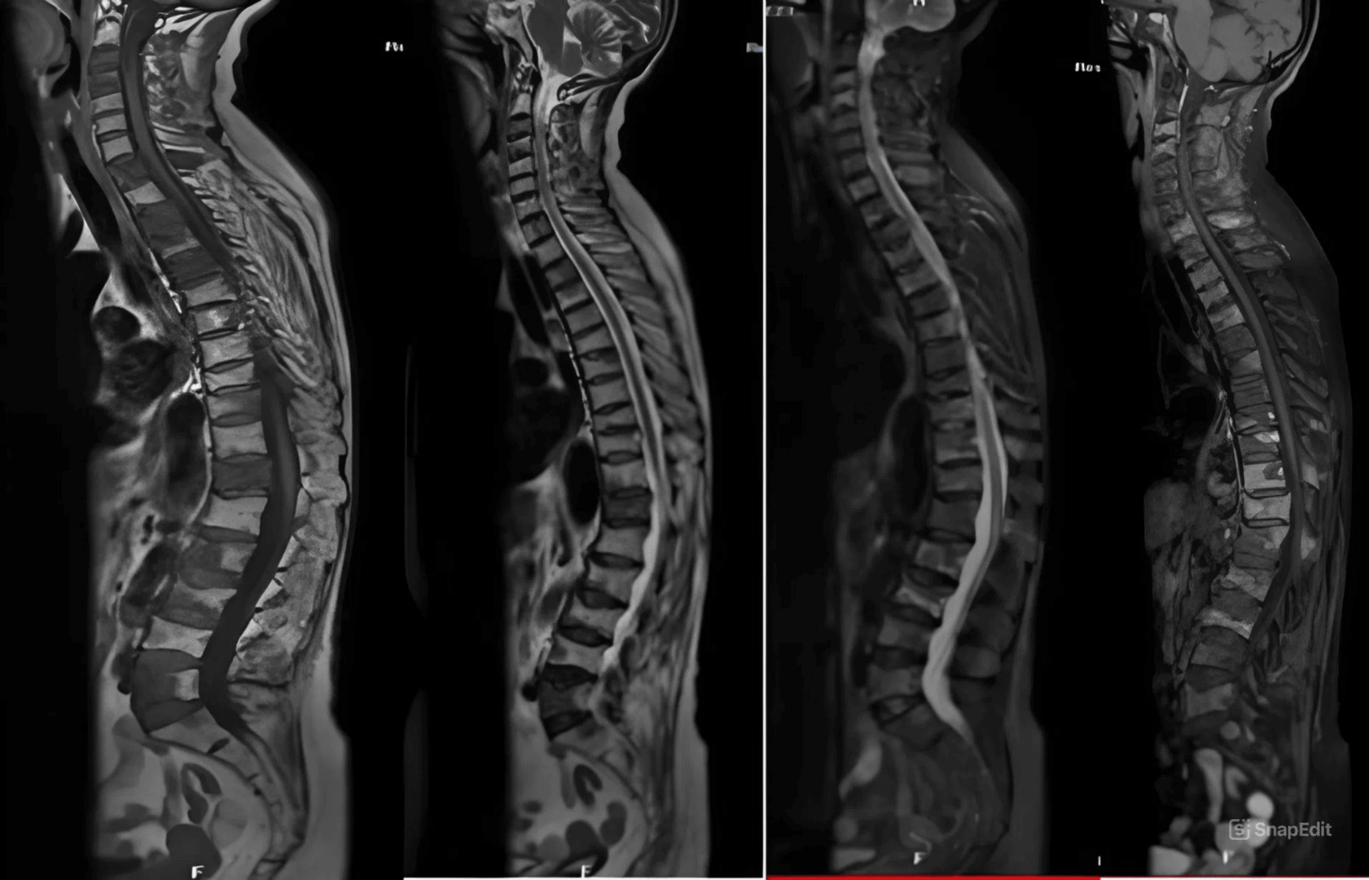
Sagittal MRI images of the spine in a patient with metastatic breast carcinoma. Multiple vertebral bodies across the cervical, thoracic, and lumbar regions exhibit hypointense signals on T1-weighted images and hyperintense signals on short tau inversion recovery (STIR), with intense post-contrast enhancement, indicating widespread vertebral metastases involving both the anterior vertebral bodies and posterior elements. Image: Courtesy of Dr. Samir Aloulou, Department of Medical Oncology, Gabes University Hospital, University of Sfax, Sfax, Tunisia.
